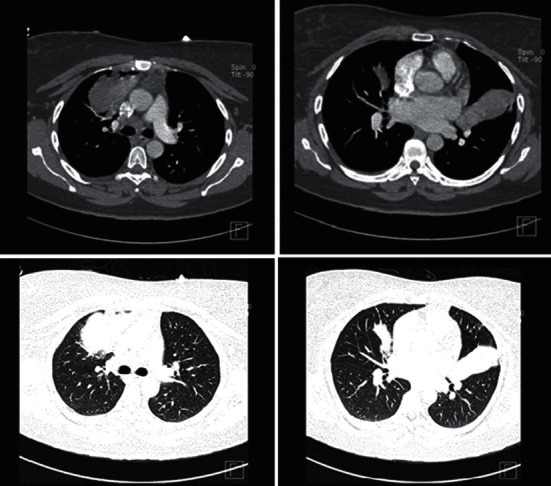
Computed tomography pulmonary angiography shows bilateral mass-like density with finger in glove sign.
